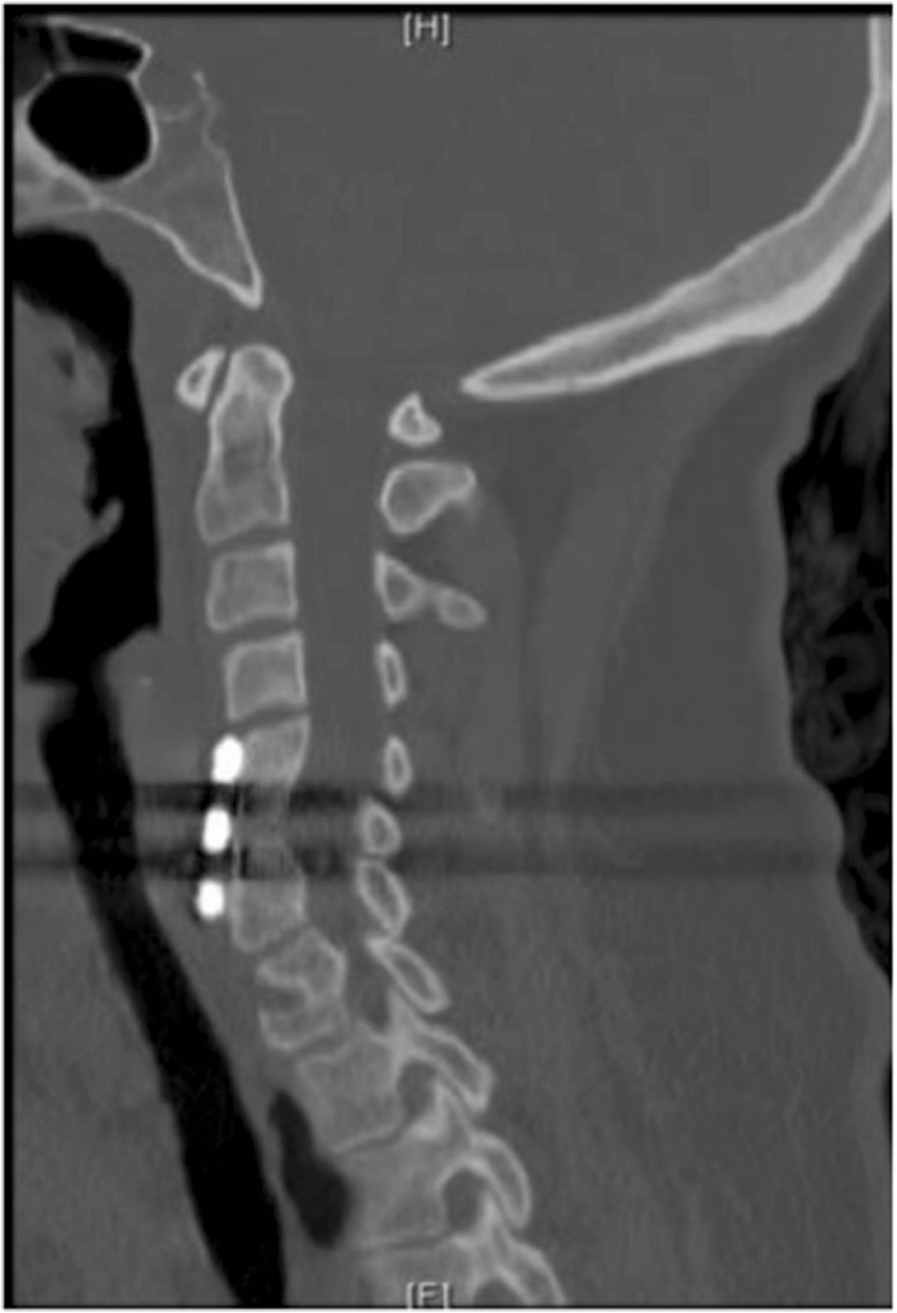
Postoperative computed tomography scan obtained at 17 months showing fusion of C5 to C7
